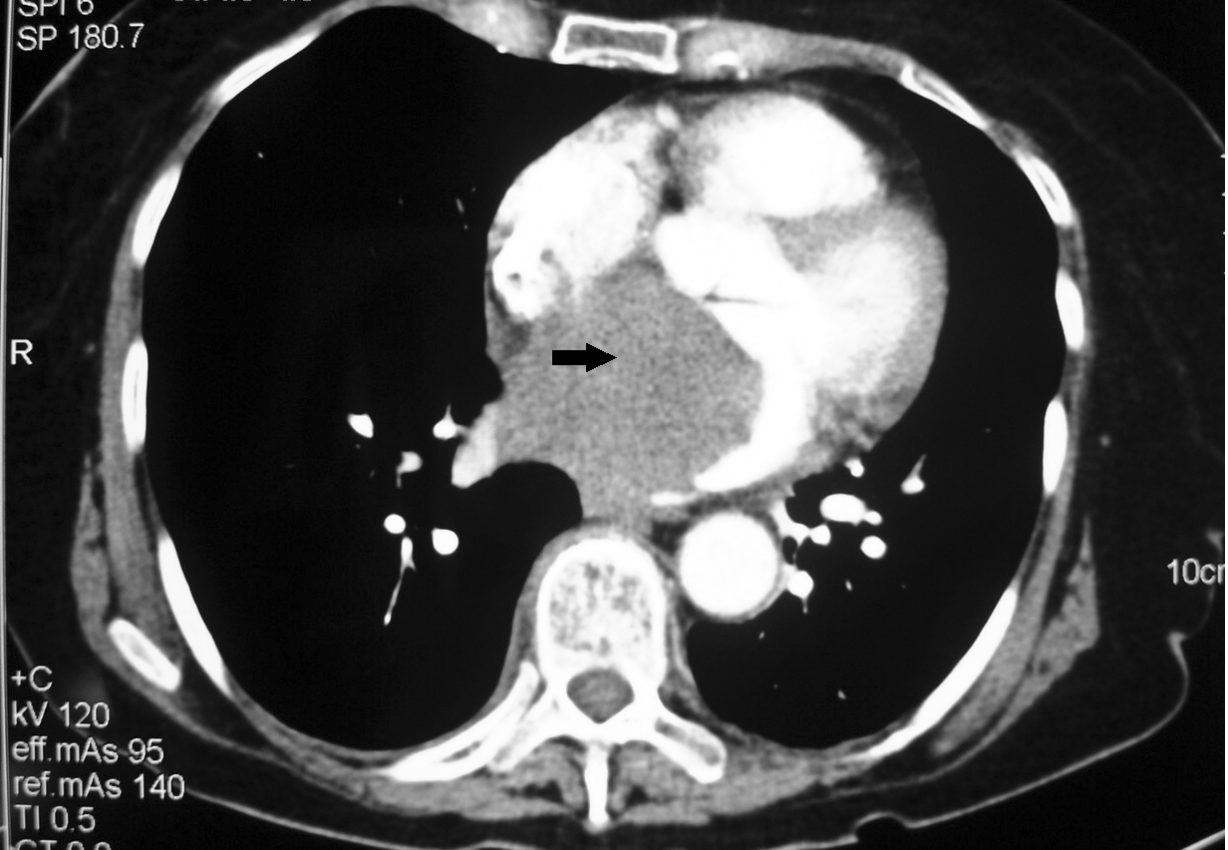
CT scan shows a large filling defect (black arrow) in the left atrium.
